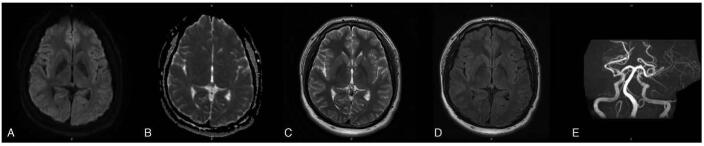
Follow-up cerebral MRI at 2 months post treatment revealed the complete resolution of cerebral edema in the left parieto-temporal lobe (A: diffusion-weighted imaging, B: apparent diffusion coefficient, C: T2-weighted imaging, D: fluid-attenuated inversion recovery). There was no change of moyamoya disease with stenotic bilateral distal internal carotid arteries, compared with previous neuroimages (E). The complete recovery of neuroimaging changes supported the diagnosis of PRES. MRI = magnetic resonance imaging, PRES = posterior reversible encephalopathy syndrome.
